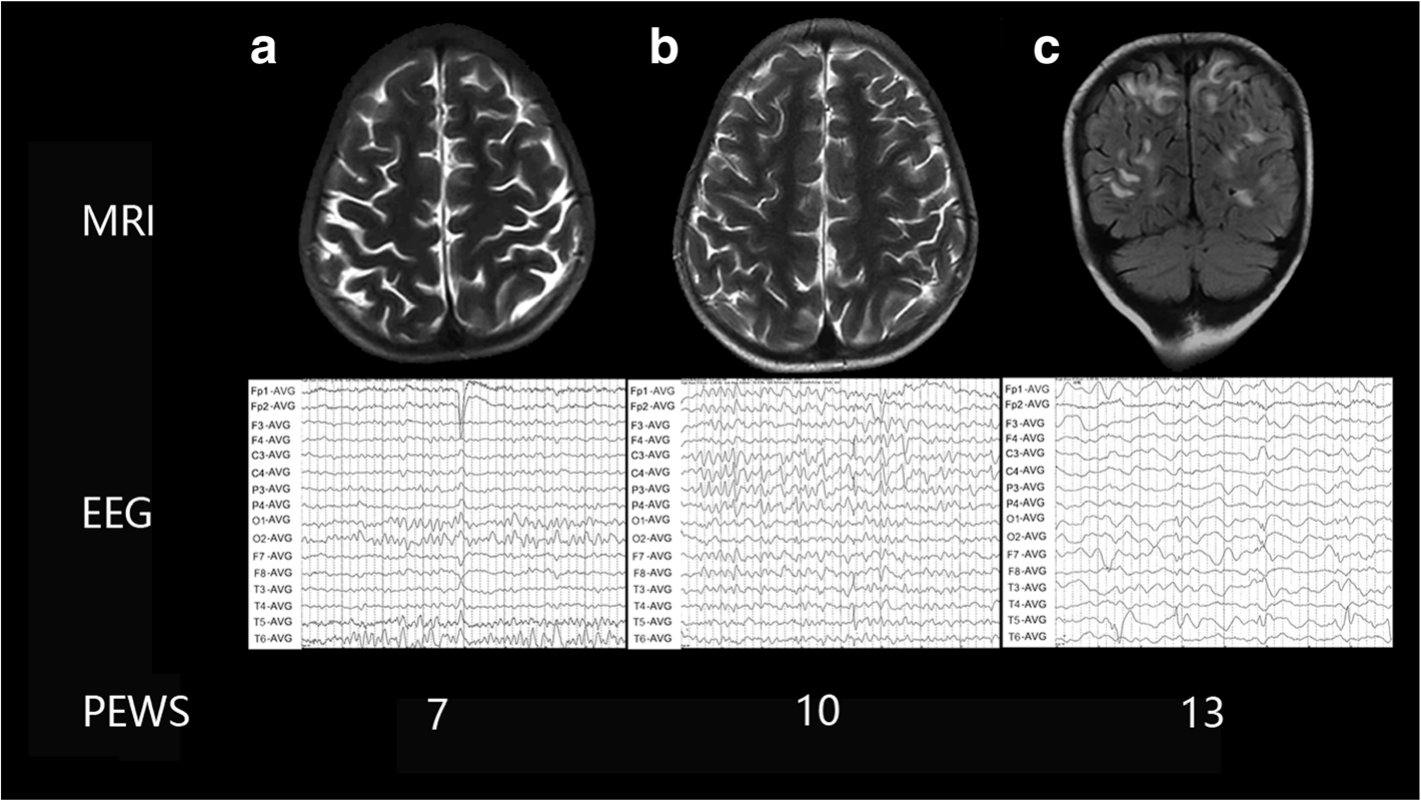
a female, five years old, EEG showed focal low amplitude slow wave, MRI was normal, PEWS 7. b female, five years old, EEG showed focal (right parietal and temporal lobe) epileptic discharge, MRI was normal, PEWS 10. c male, five years old, EEG showed slow wave background and epileptic discharge, MRI showed bilateral parieto-occipital subcortical white matter long T1 signal, long T2 signal, and equal DWI signal, PEWS 13. Abbreviations: EEG: electroencephalogram; MRI: magnetic resonance imaging; PEWS: posterior reversible encephalopathy syndrome early warning score
